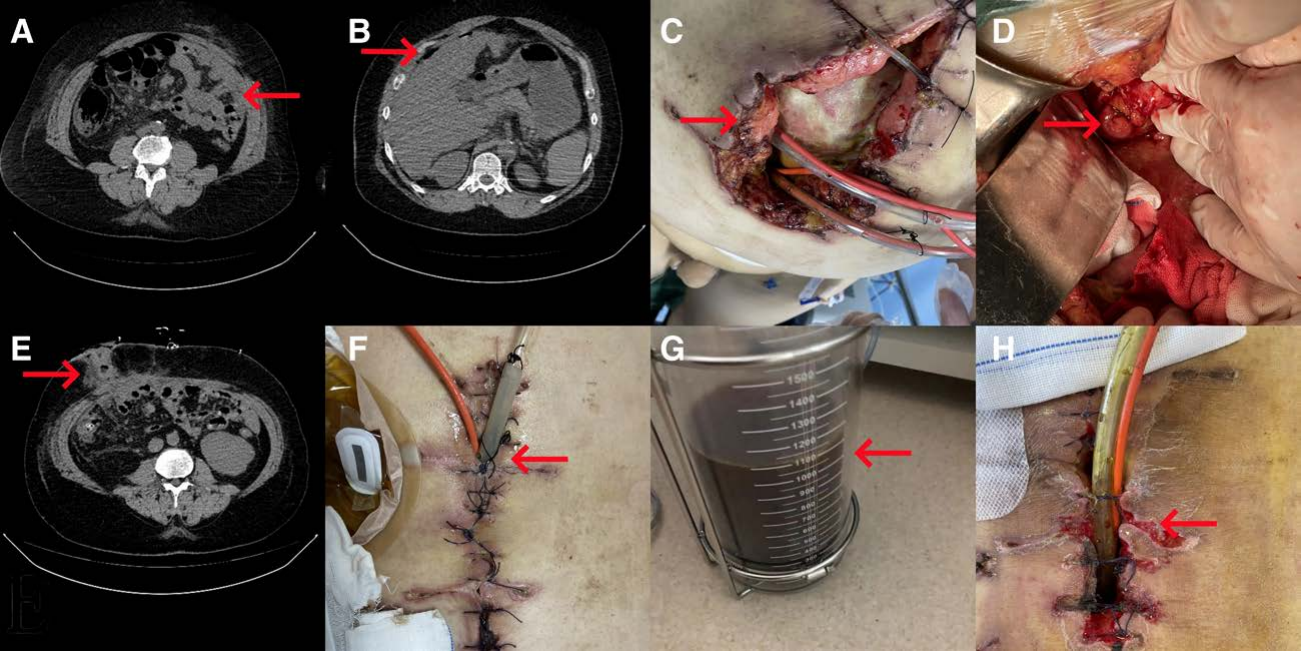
Computed tomography (CT) images and incision changes. (A) Abdominal CT revealed dense, clumped images in the abdominal wall, with some small intestinal loops gathered, indicative of the cauliflower sign. (B) Subphrenic free air was observed. (C) Postoperative abdominal infection and incisional tearing were evident. (D) Intraoperative ileal anastomotic fistula was identified. (E) Abdominal CT displayed a single ileal stoma. (F) The incision was sutured, and Li’s double cannula was placed subcutaneously. (G) Drainage from the incision exhibited turbid bloody fluid. (H) Silk cutting and necrosis of the skin margin in the incision were evident (marked by the red arrow).
